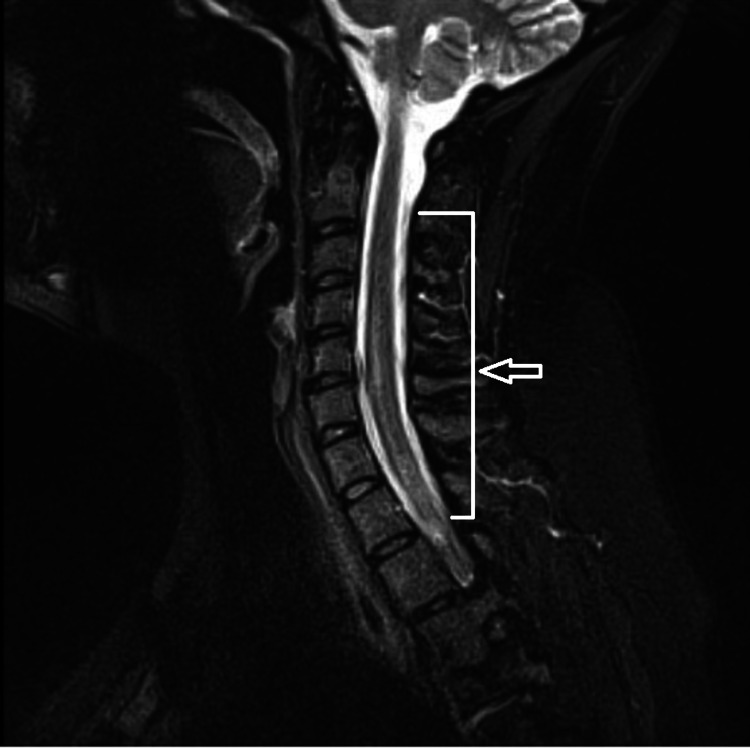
Magnetic resonance imaging of the cervical and upper thoracic spine with and without contrast showing a long segment of increased T2 signal within the central spinal cord extending at least from the C2-3 segment into the visualized thoracic spine (arrow).
